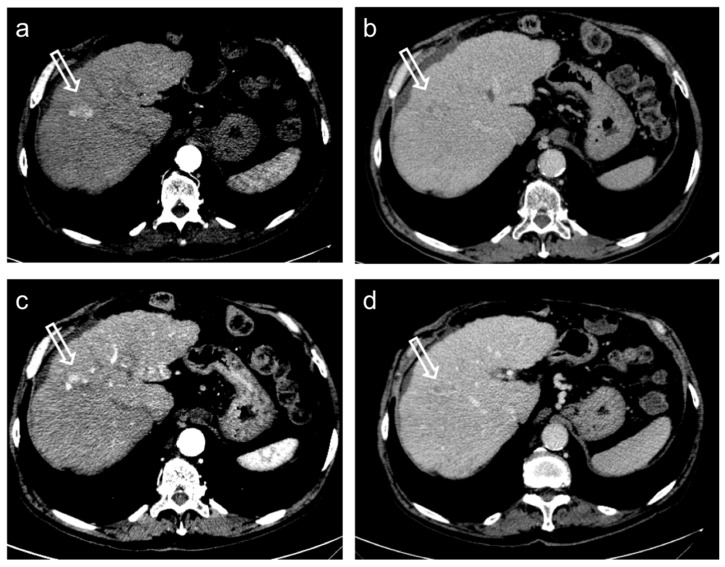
Hepatocellular carcinoma treated with transarterial chemoembolization (TACE) with partial response. Pre-treatment contrast-enhanced CT (a,b) shows a 24 mm nodule (arrow) in segment 8 with arterial hyper-enhancement (a) and late wash-out (b). After TACE (c,d), the presence of nodular hyper-enhancement in the arterial phase (arrow in (c)) with wash-out in the late phase (arrow in (d)) demonstrate the residual viable tumor consistent with a partial response to treatment.
